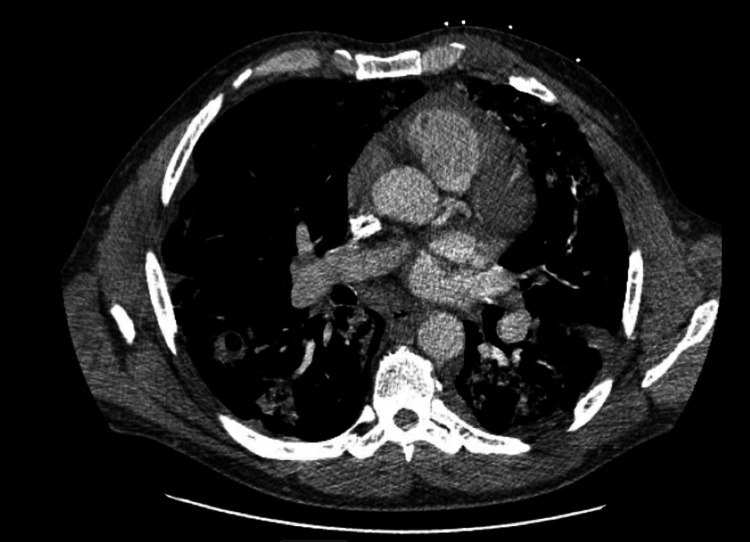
CT scan of the chest Due to the timing of the bolus, the evaluation of the pulmonary arteries is limited. No emboli were found in the central or lobar pulmonary arteries, and the main pulmonary artery is dilated. The scan showed no significant abnormality in the thoracic aorta or heart. There is no coronary artery calcification, but there are prominent reactive mediastinal lymph nodes. The lungs show worsening bilateral consolidations and ground-glass opacities, along with a cavitating lesion in the right lower lobe
